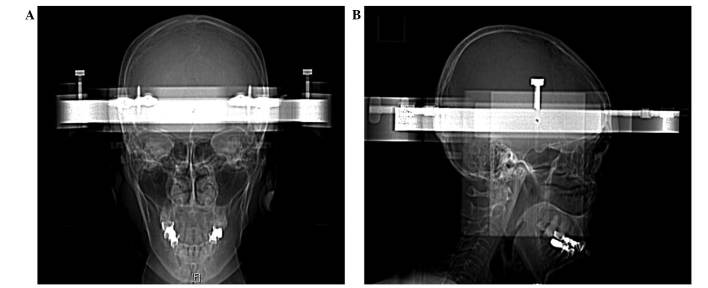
Computed tomographic images showing the setup for stereotactic aspiration. (A) Antero-posterior view; (B) lateral view.
